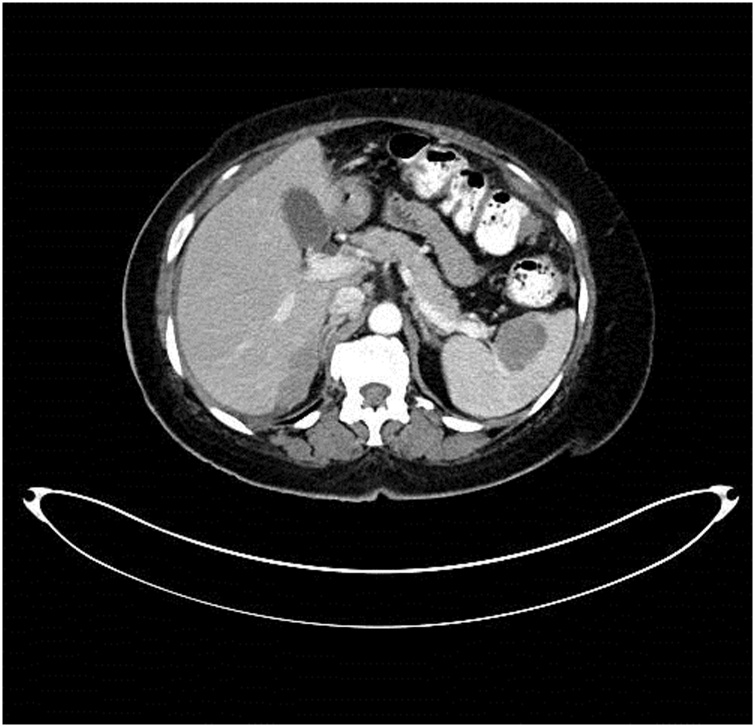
This 59-year-old woman presented with abdominal pain and an enlarging right ovary. Peritoneal metastases were present beneath the right hemidiaphragm and within the pelvis. A 3.8 cm mass was located within the splenic parenchyma and identified by the radiologist as “splenic metastases”.▪Biopsy of the abdominal wall mass revealed adenocarcinoma likely of gynecologic origin.▪Because of the large extent of disease and the presumed hematogenous metastases to the spleen, neoadjuvant chemotherapy with carboplatin and paclitaxel was recommended. Four cycles were planned. Pretreatment CA125 tumor marker was 1283 units/mL.▪In January 2020, preoperative evaluation revealed significant improvement of abdominal discomfort. CA-125 had decreased to 39.1 units/mL. Repeat CT scan showed reduction of tumor volume at all sites but the disease had persisted. The splenic metastasis had responded to the systemic chemotherapy but was still visible by CT (Fig. 2).
